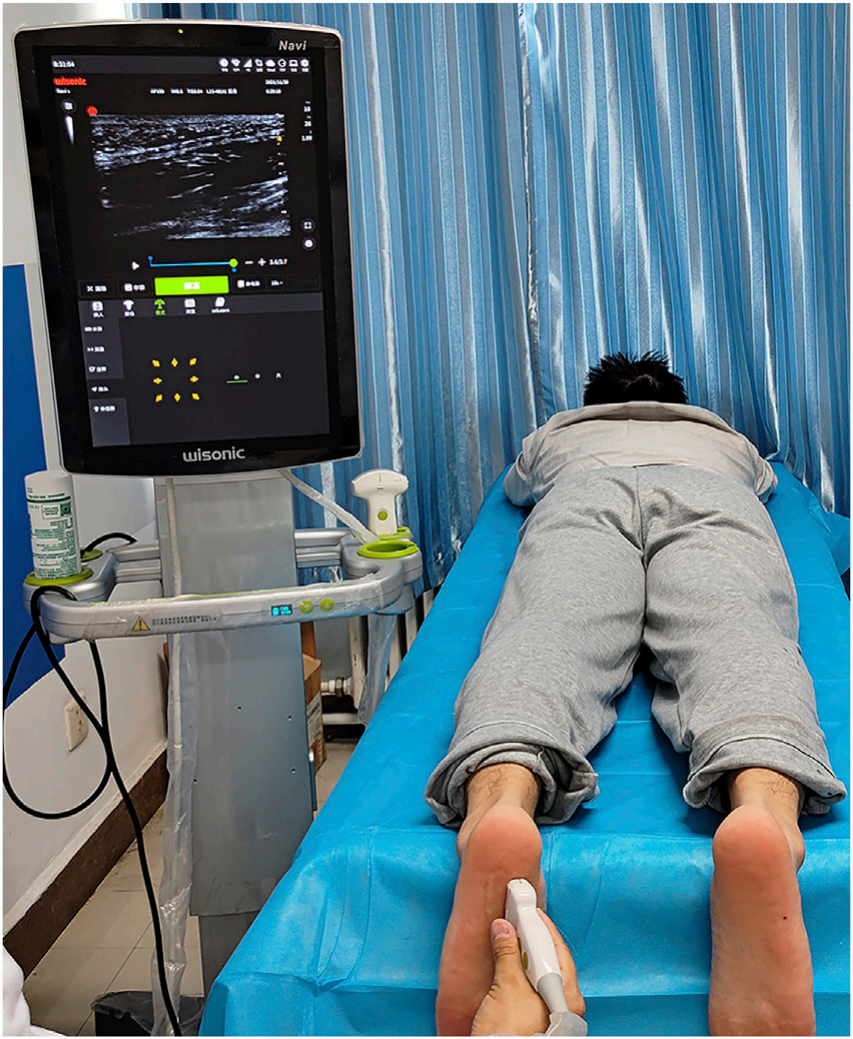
Position for ultrasound measurement of plantar fascia angle.
